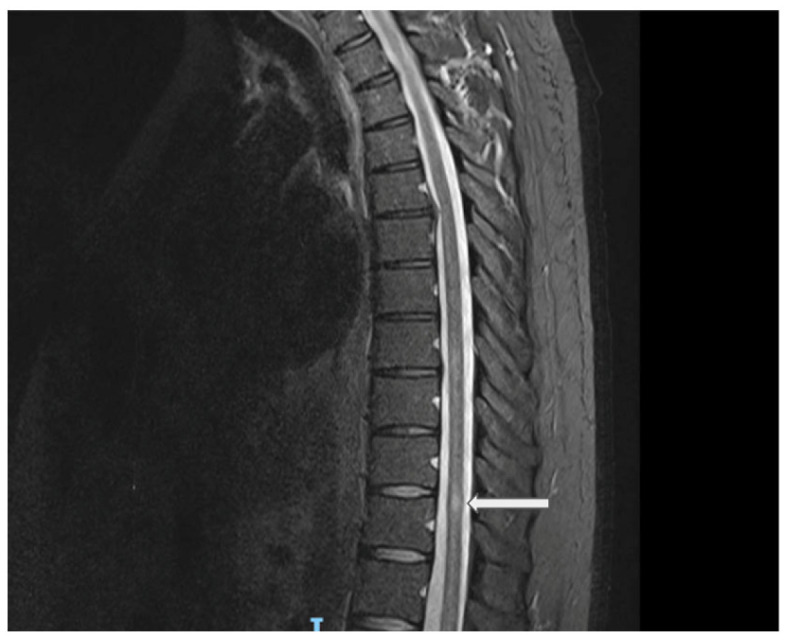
Sagittal spine MRI (STIR sequence) showing a central thoracic cord lesion with high T2 signal intensity opposite the superior aspect of the T11 vertebral level, associated with subtle cord expansion. Explanation of the arrow figure: central thoracic cord lesion with high T2 signal intensity opposite the superior aspect of the T11 vertebral level.
